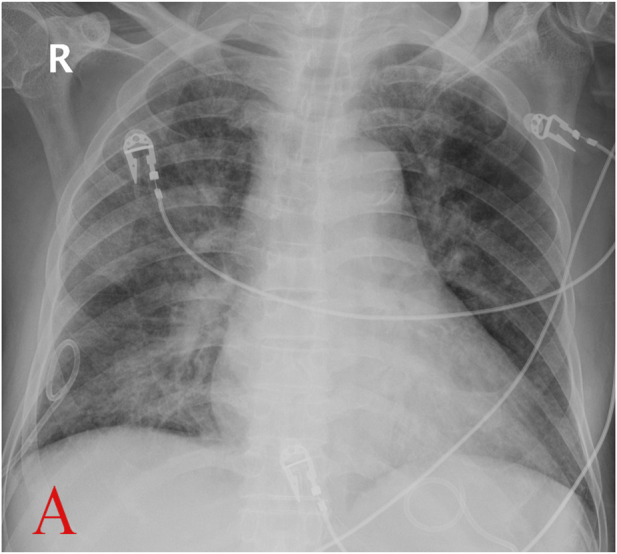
Chest X-ray before extubation (A, Apr.14) (with permission from the patient.)
